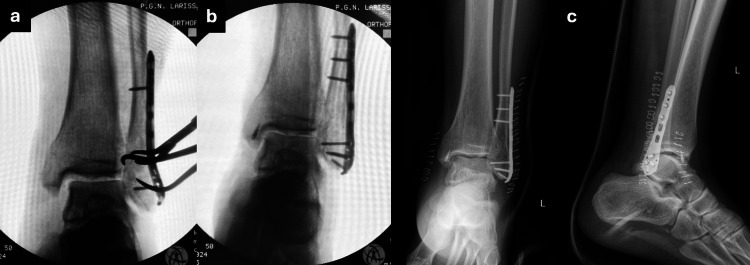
Intra-operative and post-operative X-rays. (a) Intraoperative radiological examination revealing medial joint widening despite fibula reduction (b) Complete restoration of ankle joint congruency after disengagement of the deltoid ligament which was reattached on the medial malleolus using a suture-anchor (c) Post-operative anteroposterior and lateral X-rays.
